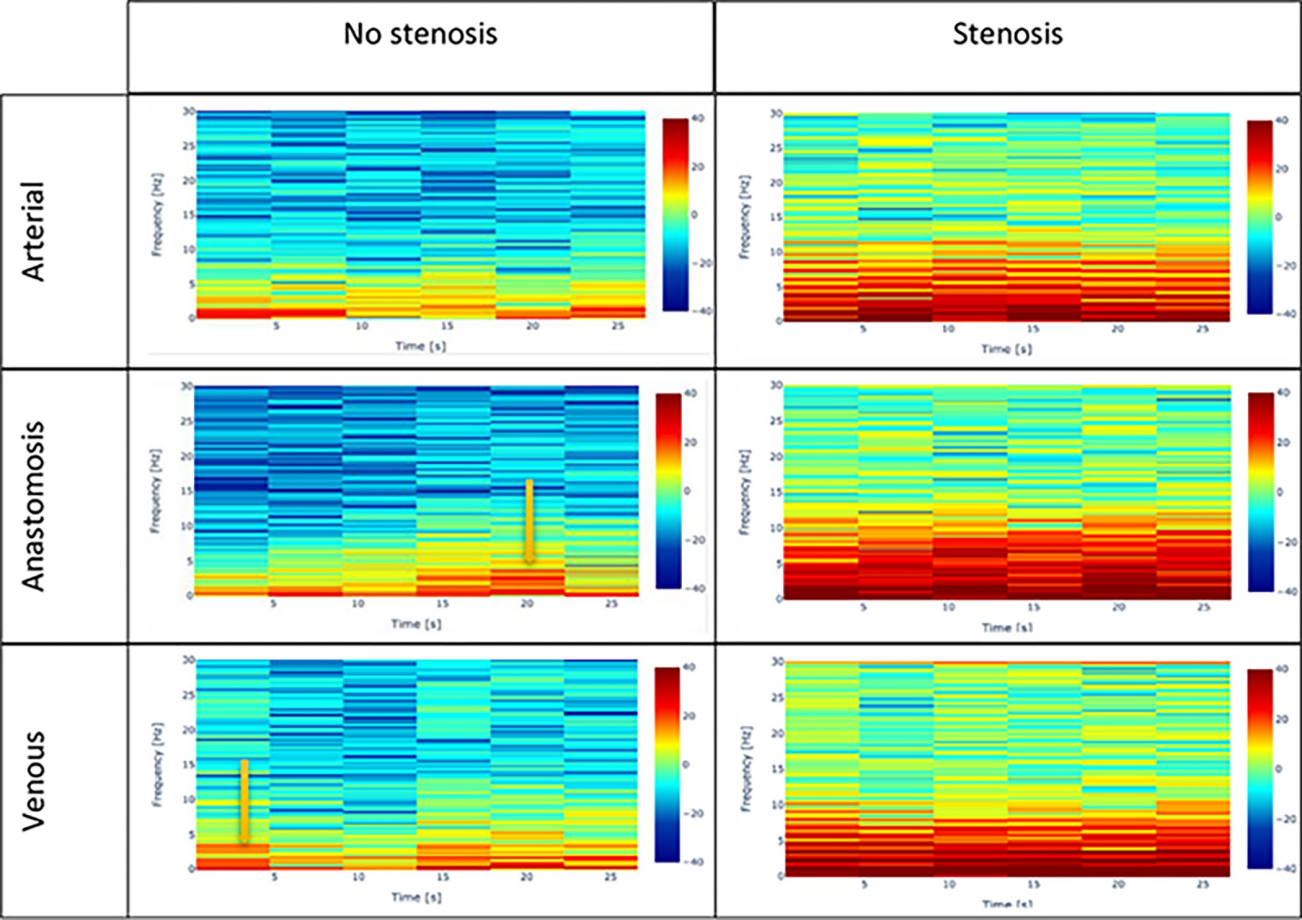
Spectrograms of blood flow only with and without stenosis. The spectrograms show the signals picked up in the blood flow only of arterial inflow, anastomosis, and venous outflow of fistulae with stenosis and no stenosis. The Doppler readings in the arterial with no stenosis are PSV (197 cm/s)–VF (1721 ml/min)–diameter (7 mm) and with stenosis are high resistive PSV (368 cm/s)–VF (290 ml/min)–diameter (5.6mm). In the anastomosis with no stenosis are HH 07 –PSV (250 cm/s)–diameter (3.2 mm) and with stenosis are PSV (340 cm/s)–diameter (2.9 cm)–stenosis (40–50%). In the venous with no stenosis are HH 04 –PSV (100 cm/s)–VF (610 ml/min)–diameter (4–9 mm) and with stenosis are PSV (693 cm/s)–VF (490 ml/min)–stenosis (45–50%). The *purple arrows* show the random peaks with high intensity, which correspond to an artefact either caused by the patient movement or by the operator moving transducer out of the plane.
